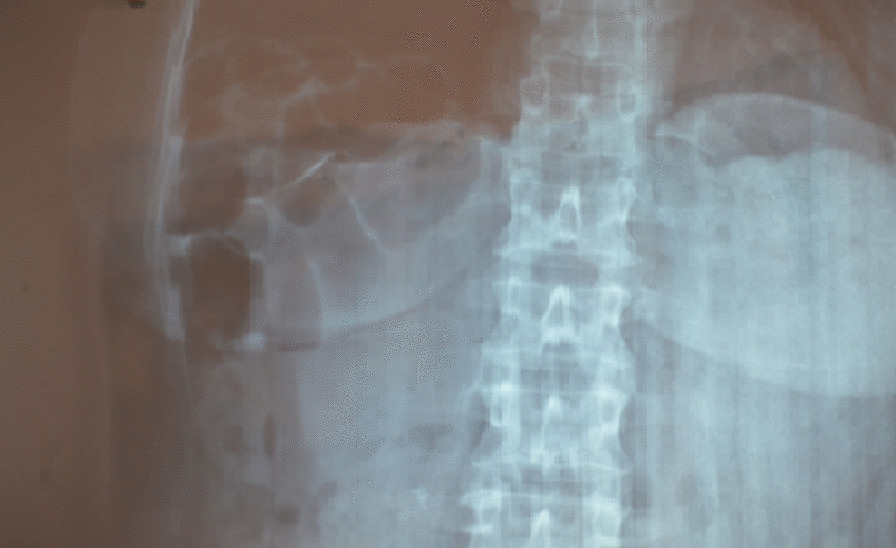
X-ray, loops of small bowel into the right part of the thorax
